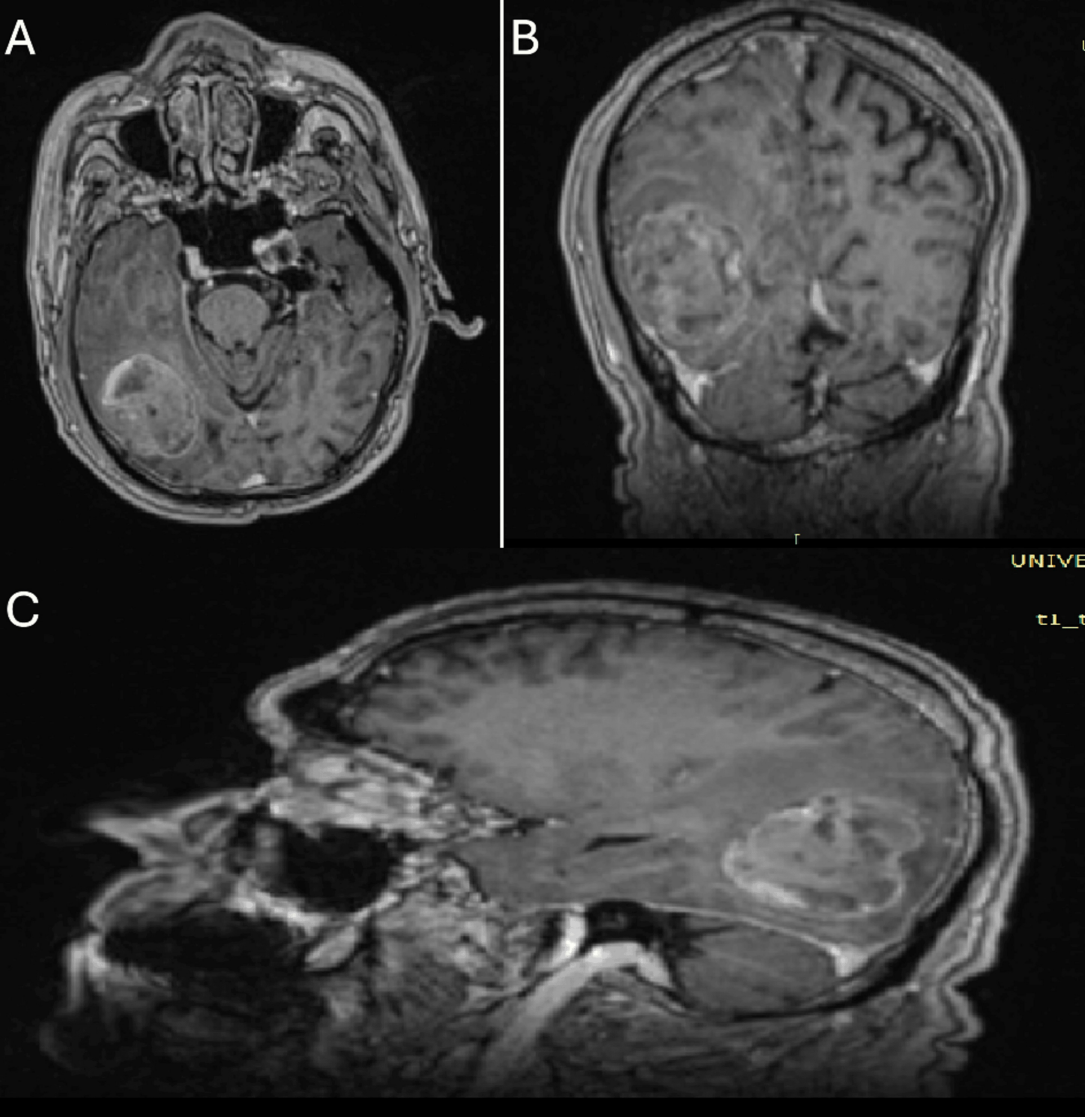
Pre-operative MRI T1-weighted image + Gd The pre-operative magnetic resonance imaging (MRI) study, performed with a gadolinium-based contrast agent, demonstrated a lesion exhibiting peripheral enhancement. The upper left image corresponds to an axial view (A), the upper right image represents a coronal view (B), and the lower image depicts a sagittal view (C).
